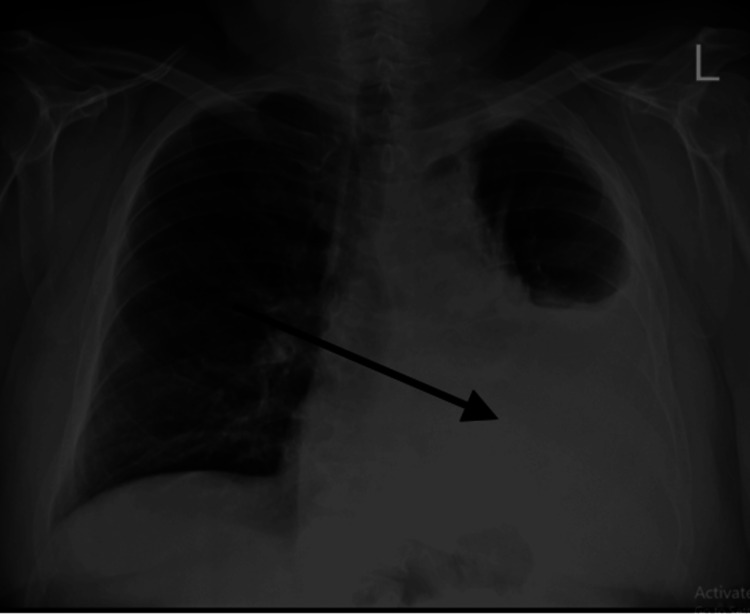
Chest X-ray showing an extensive lung mass compressing the left lung.
